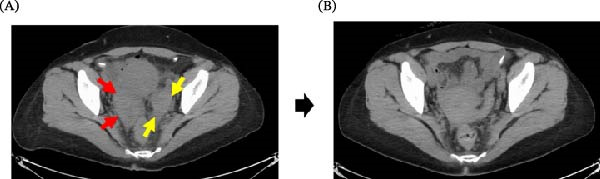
Perioperative computed tomography images of the pelvis in Case 5. (A) The findings 1 week before surgery. (B) The findings 1 month after surgery. The yellow arrow indicates the enlarged left ovary, and the red arrow indicates the enlarged right ovary.
